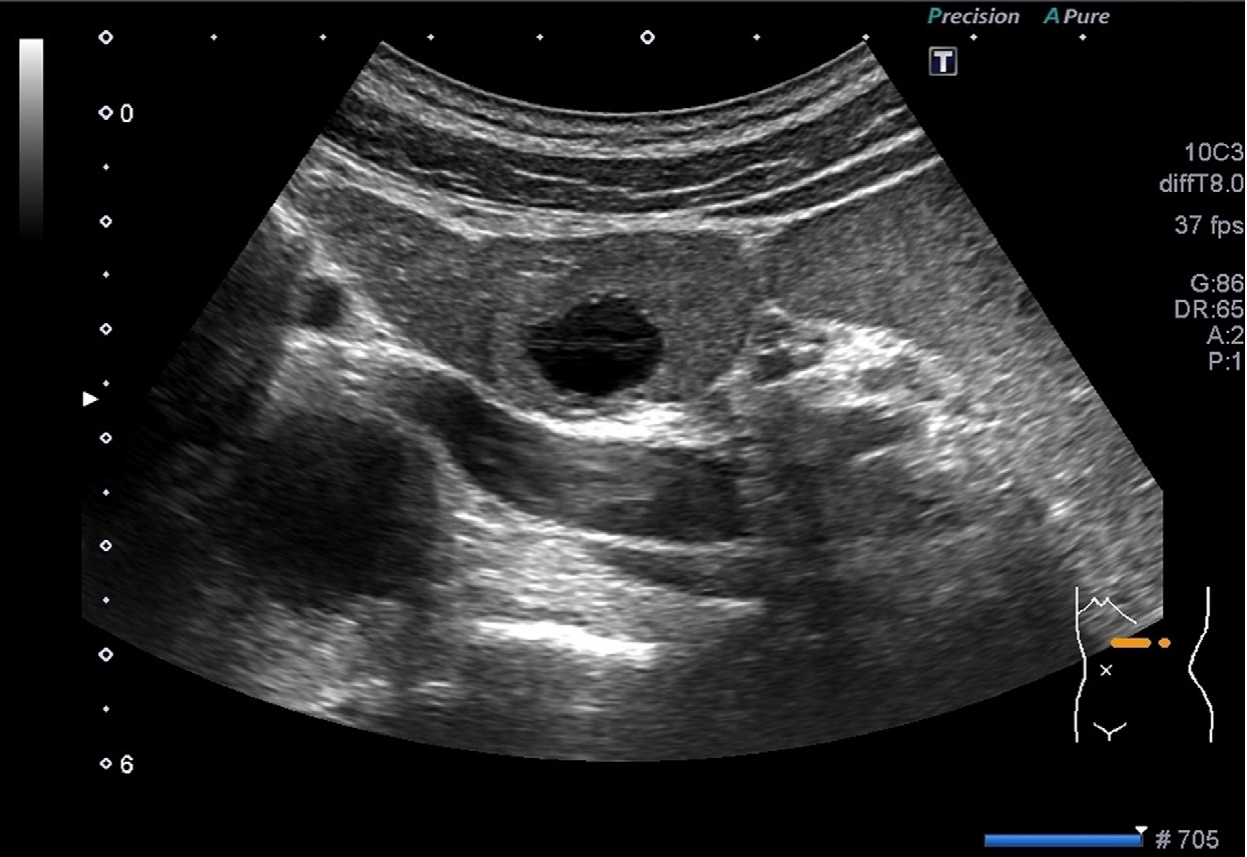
Abdominal ultrasonography revealed a 25‐mm cystic tumor located in the pancreatic tail.
